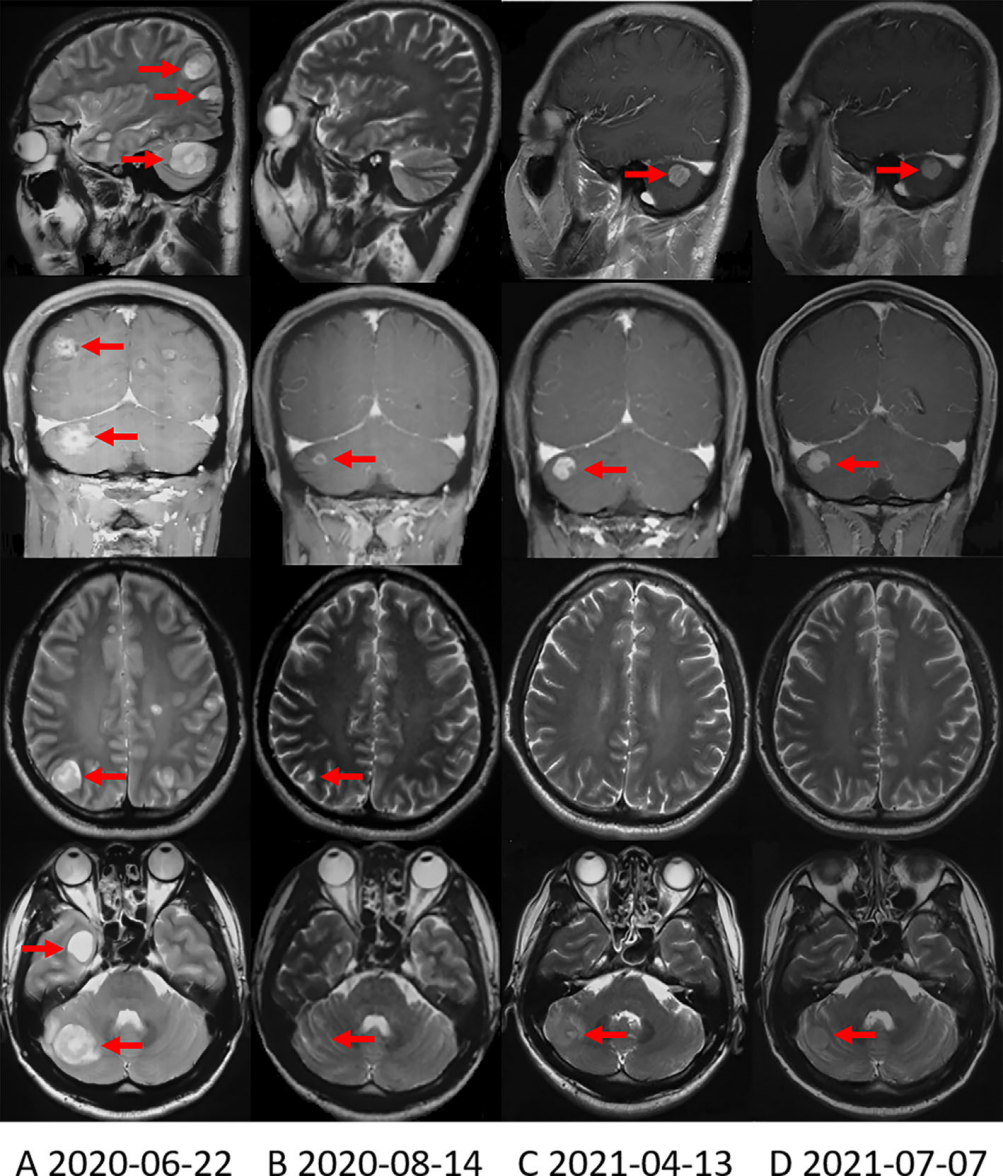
Brain MRI shows the change in brain metastases with treatment. (A) Brain MRI when the brain metastases first appeared. (B) Brain MRI showed that the brain metastases were significantly reduced after applying alectinib for nearly 2 months. (C) On April 13, 2021, Brain MRI showed that the right cerebellum lesion increased in size. (D) On July 7, 2021, the most recent Brain MRI showed that the brain metastases were stable.
